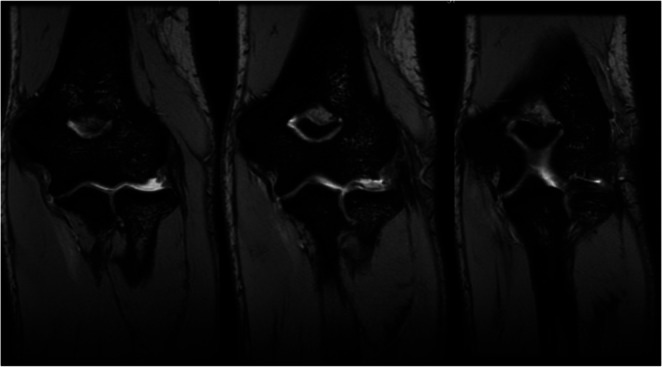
Coronal T2 MRI series demonstrating a distal tear of the ulnar collateral ligament
